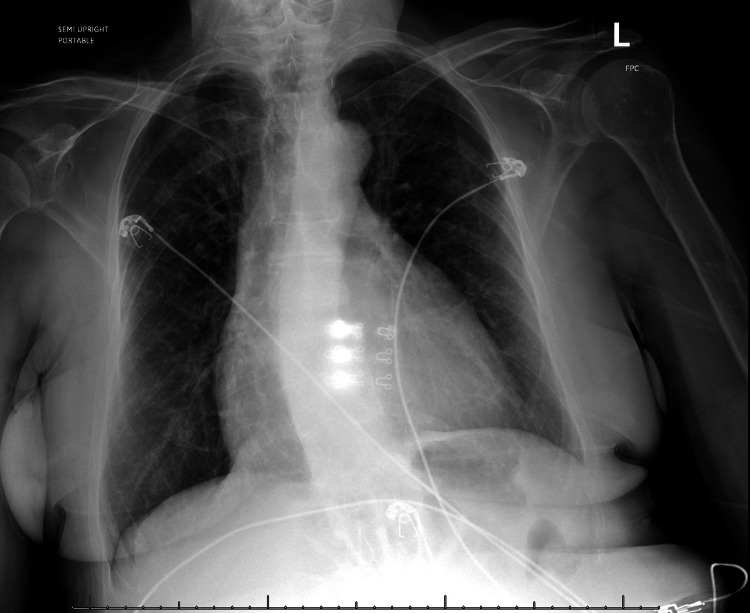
Normal chest X-ray The image depicts a normal chest X-ray without any significant findings
